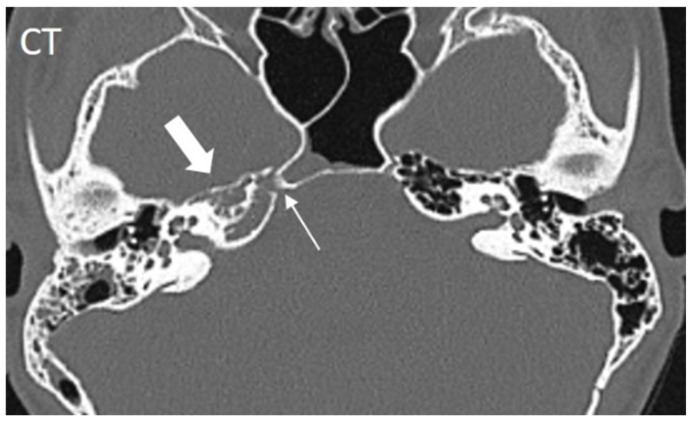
CT of a young patient with clinically present Gradenigo syndrome. It shows the lytic destruction of the bone trabeculae of the petrous apex as well as its obliteration without expansion (thick arrow). The course of the abducens nerve in the Dorello canal through the affected petrous apex can be visualized (thin arrow).
